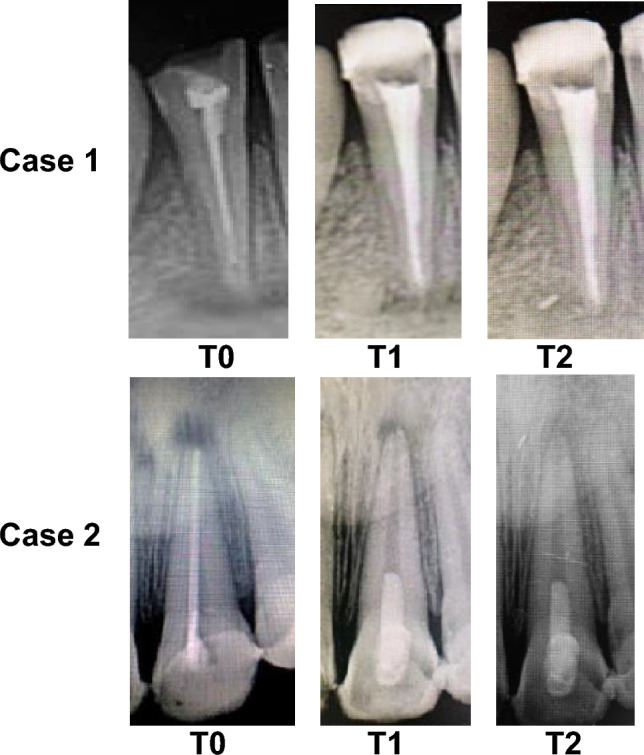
Periapical radiographs of two cases at the follow-up intervals (T0: preoperative, T1: after 6 months, and T2: after 12 months) shows the progress of healing of the periapical radiolucency. Case (1) represented a 13-year-old male. Tooth #41 has retreated with non-surgical endodontic treatment. Case (2) represented an 11½-year-old female. Tooth #21 was treated with REPs. At T1 and T2, both teeth showed radiographic success.
